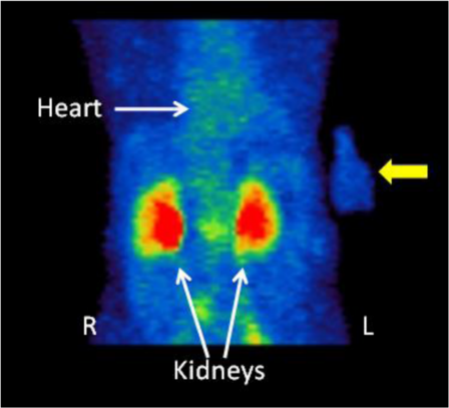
A MIP image from the initial SPECT study (0.5 h after administration of the therapy commenced) for one subject is shown, indicating the location of the calibration flask (*yellow arrow*) relative to the subject. The kidneys (*red*) are seen to be the organs with the highest uptake at this imaging time point. Subject’s right side (R) and left side (L) are indicated
